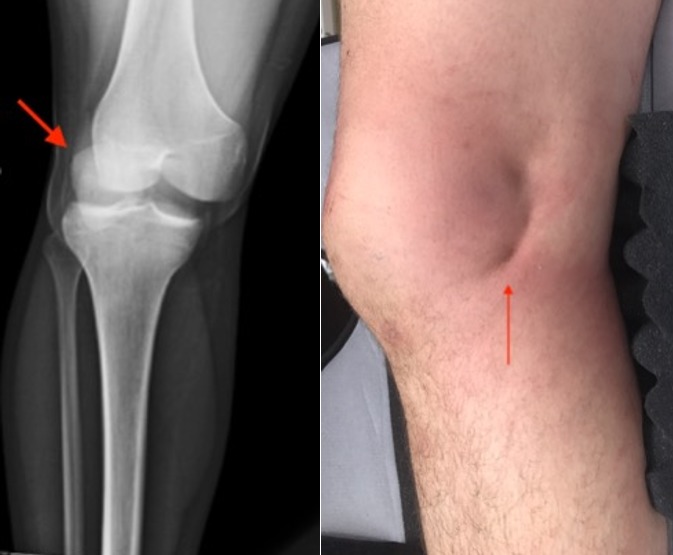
Xray showing posterolateral dislocation of the knee. Note the maintained alignment of the patella and the tibia with respect to the femur; pucker sign noted medially
